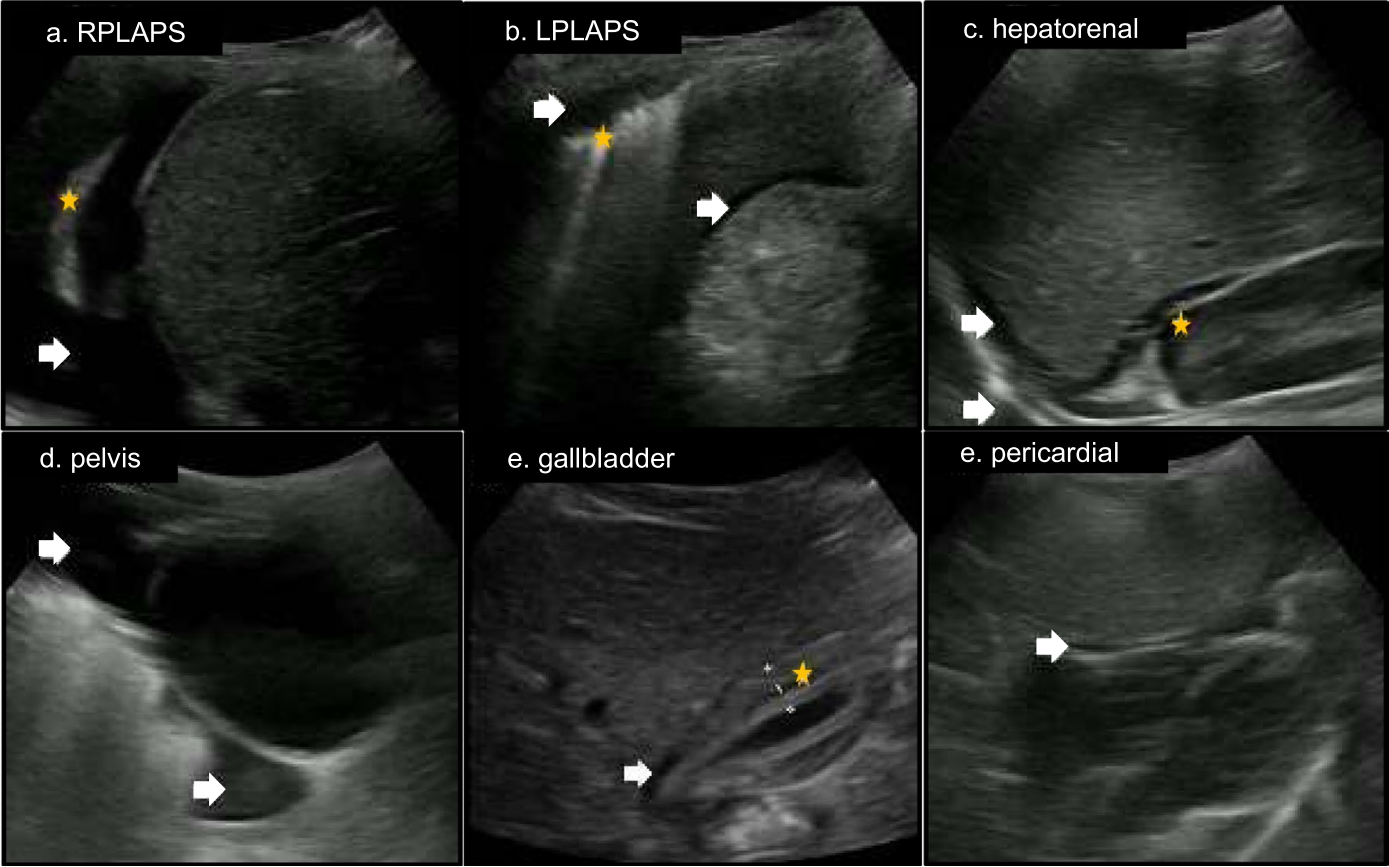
Point-of-care ultrasound findings suggesting plasma leakage. a RPLAPS right posterolateral alveolar or pleural syndromes-point demonstrating pleural effusion (arrow) and lung atelectasis (star); b LPLAPS left posterolateral alveolar or pleural syndromes-point demonstrating lung atelectasis (star), pleural effusion (left arrow), and splenorenal ascites (right arrow); c hepatorenal ascites (top arrow), right pleural effusion (bottom arrow), and perirenal fluid (star); d pelvis demonstrating abdominal ascites (top arrow) and liquid in rectovesical pouch (bottom arrow); e gallbladder with calipers measuring gallbladder wall (star) thickness and demonstration of pericholecystic fluid (arrow); f pericardial effusion (arrow)
